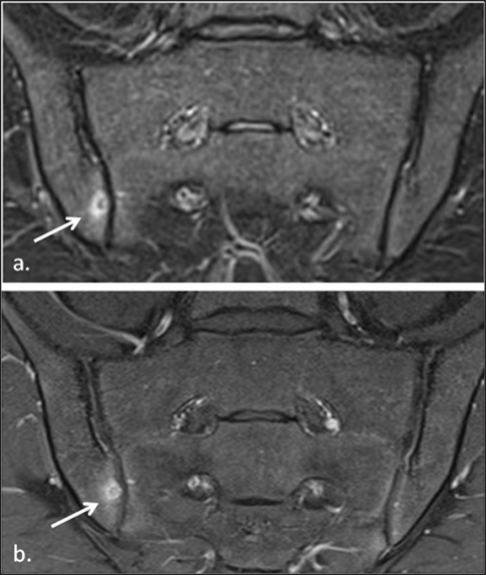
Active sacroiliitis in a 17-year-old girl with JSpA according to global assessment as well as to the ASAS definition of a positive MRI for sacroiliitis. a. Semicoronal STIR image shows an active lesion with BME at the iliac side of the right sacroiliac joint on two consecutive slides (only one slide shown) (arrow). b. Corresponding semicoronal fat-saturated T1-weighted image shows vivid enhancement of this site (arrow).
